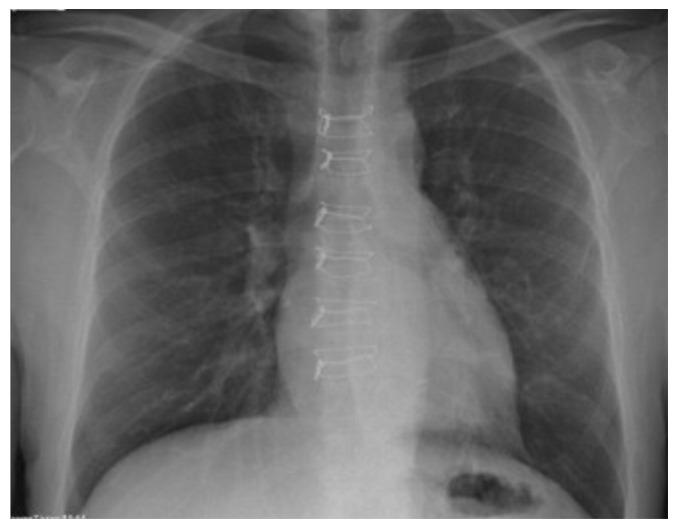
X-ray image: sternal closure using the “butterfly” technique on chest.
